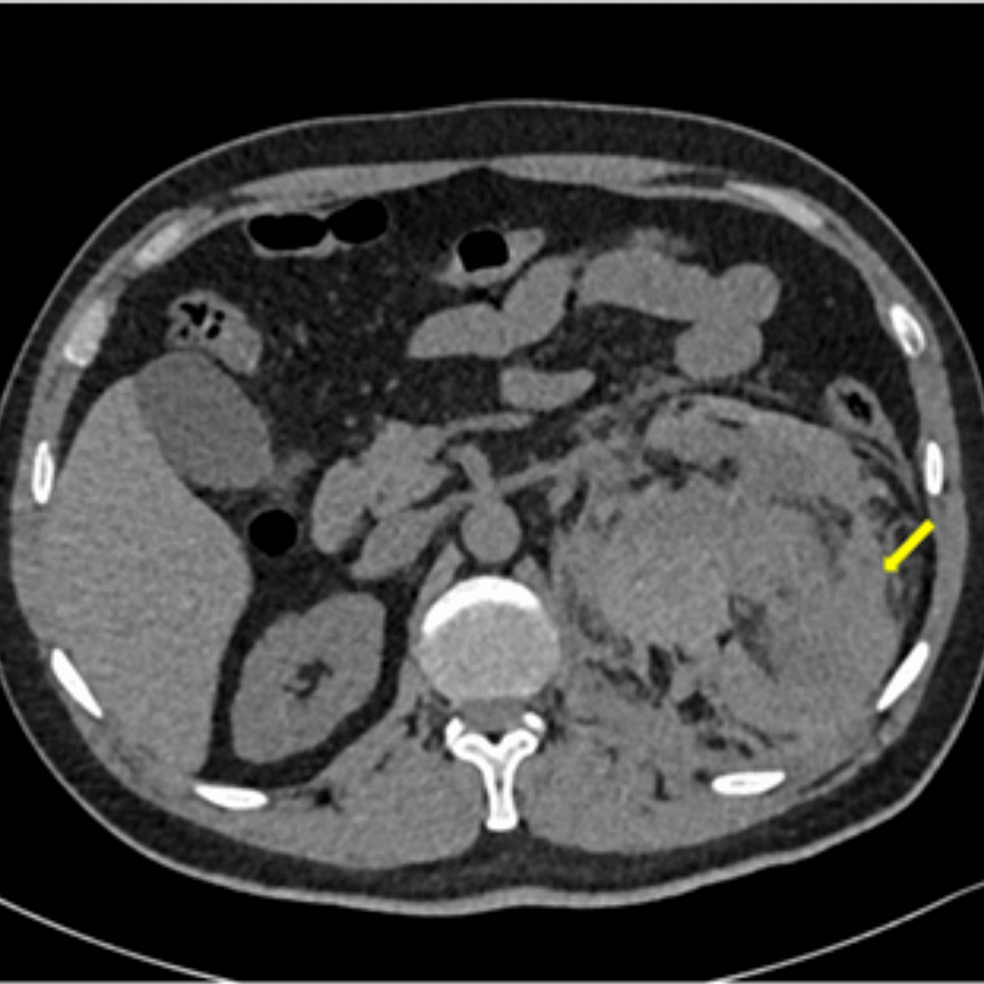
CT urinary tract: axial cuts showing large retroperitoneal hematoma in the left perinephric space extending caudally to the left lower abdomen (yellow arrows).
